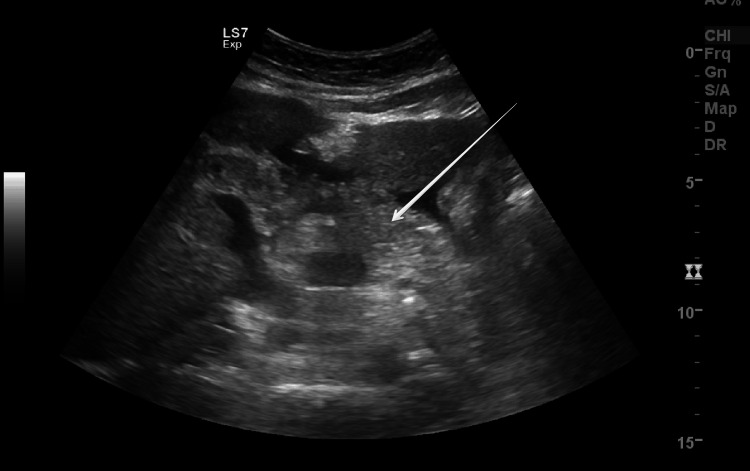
Ultrasound image shows pancreatic edema.
